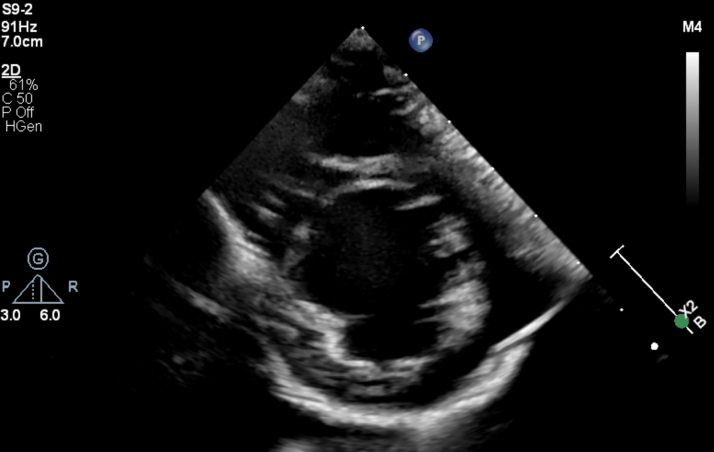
Most Recent Echocardiogram Echocardiogram (parasternal short axis view) from most recent follow-up, approximately 15 months after hospitalization.
